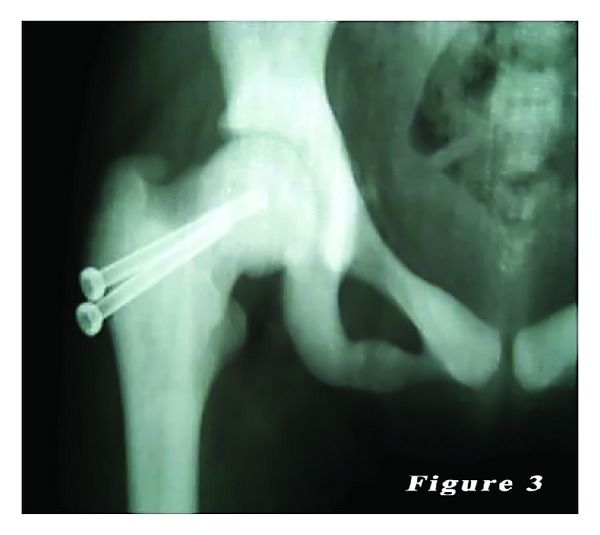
Her last radiography. It looks like fracture union.
